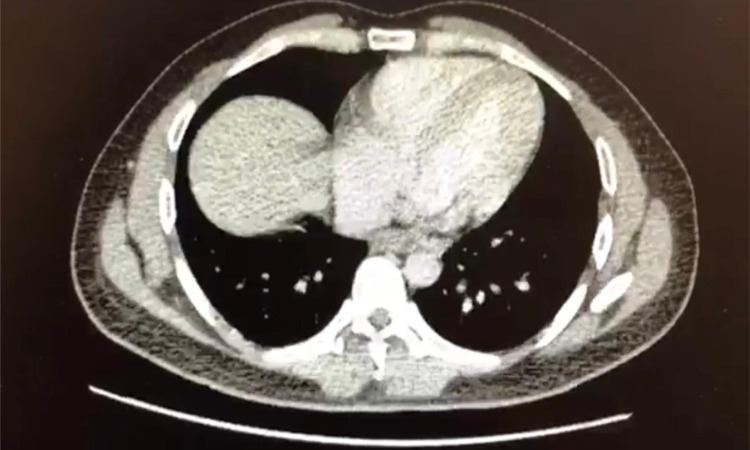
CT chest image showing bilateral segmental pulmonary arterial emboli
